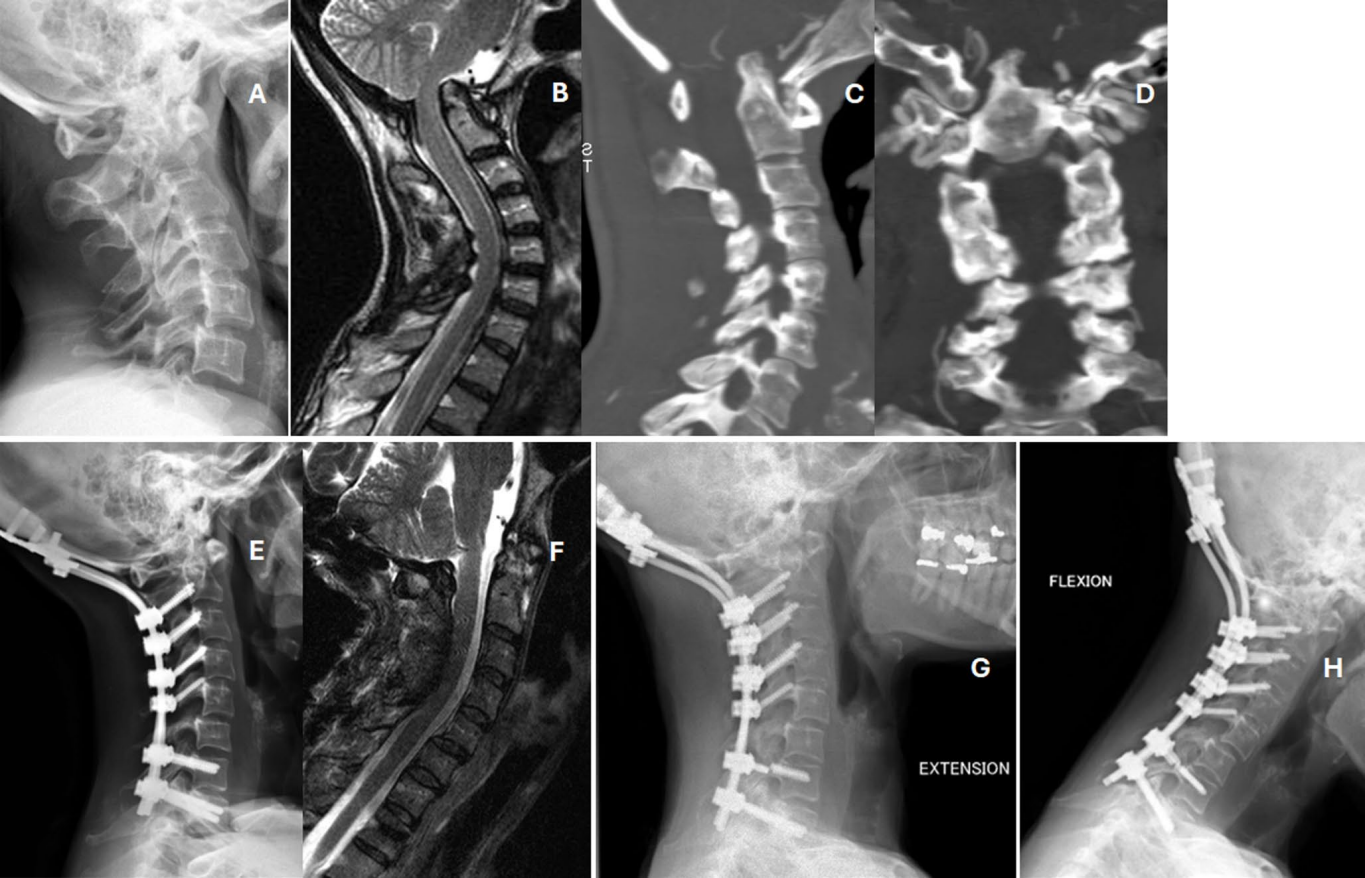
Case 11 Table 3. A: 50-year-old female with vertical settling, fixed flexion deformity and non-reducible atlanto-axial dislocation in flexion-extension radiographs. Also combines a C5-C6 subluxation. Neurological status Ranawat IIIB; B: MRI shows cranial displacement of the odontoid process with brainstem compression; C and D: Sagittal and coronal angio-CT scan. Odontoid process is prominent into the foramen magnum; E and F Postoperative XR and MRI showing correction of the deformity and indirect neurological decompression due to deformity correction—no laminectomy- (2011); G and H: Flexion-extension radiographs in November 2024, broken screws at C7 five years ago
